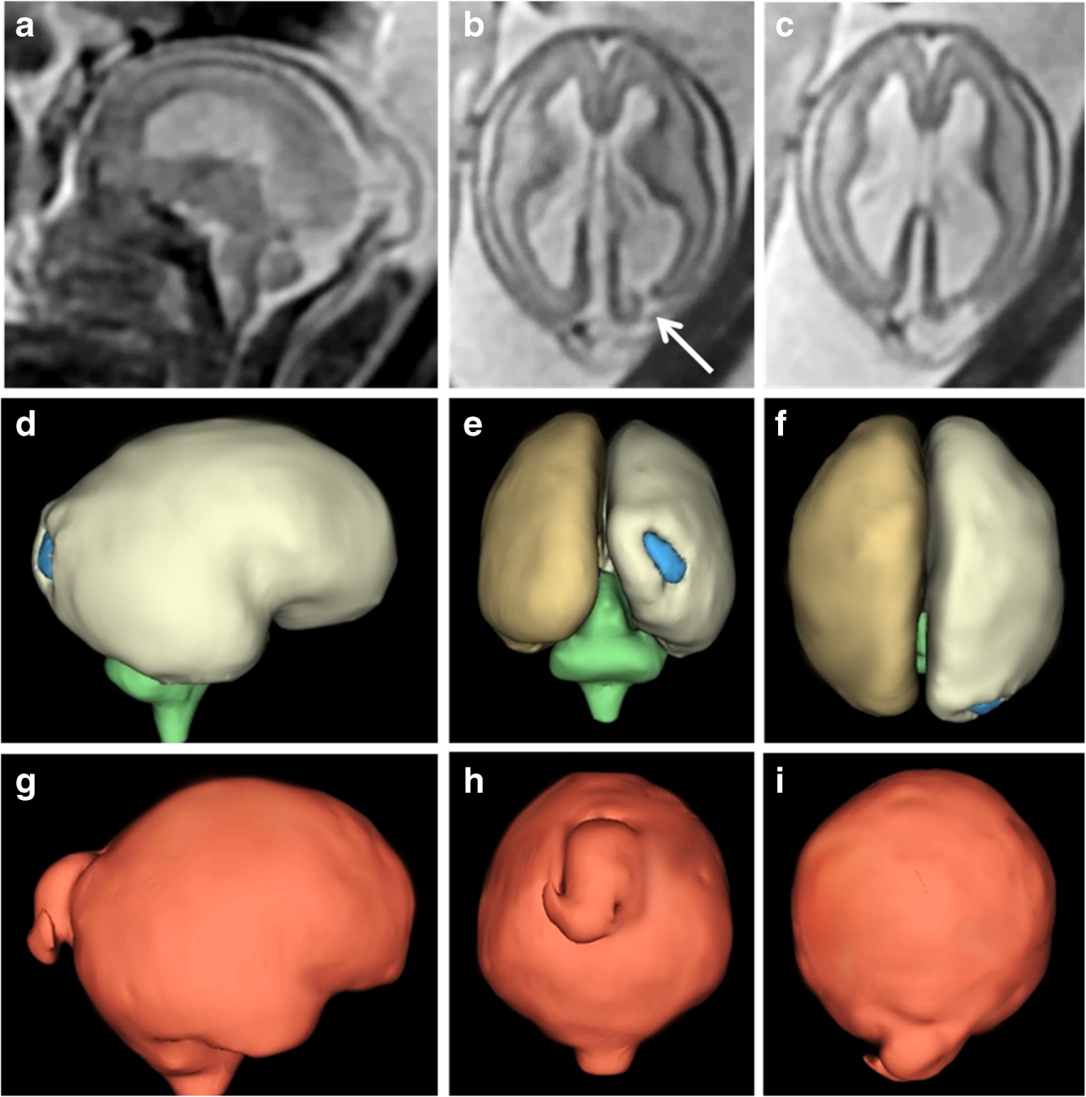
Unilateral schizencephaly (type 2) in a 21gw fetus. Sagittal (a) and axial (b, c) ultrafast T2-weighted images show a cleft in the right occipital lobe with opposed deep portions (arrowed on b). Subependymal heterotopia was also present (not shown). Those images are reversed to be consistent with the constructed model of the brain (d—right lateral, e—posterior and f—superior) which show the superficial part of the cleft is quite wide. There is a midline meningocoele posteriorly which is well shown on the models of the external CSF spaces (g–i)
